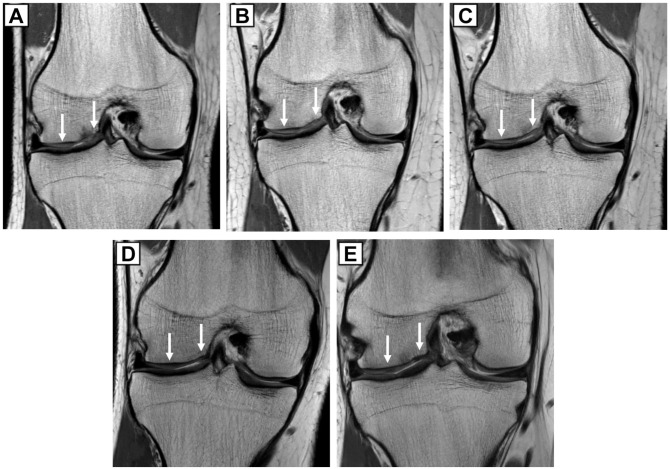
Proton density fast spin echo magnetic resonance imaging of (A) a chondral defect preoperatively on the lateral femoral condyle (between white arrows) as well as the matrix-induced autologous chondrocyte implantation (MACI) graft at (B) 1 year, (C) 2 years, (D) 5 years, and (E) 17 years after surgery.
